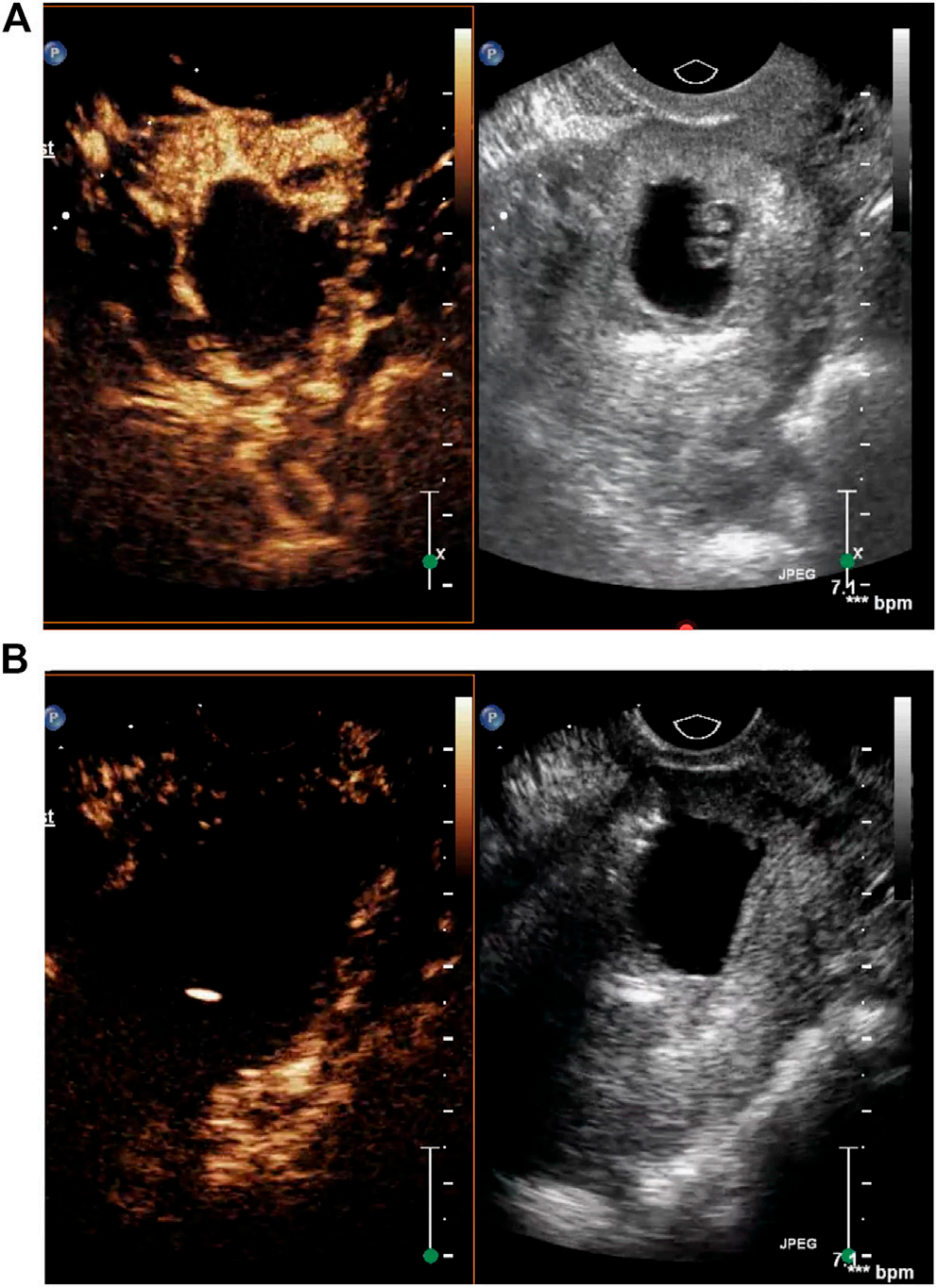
(A) The contrast-enhanced ultrasound picture indicated abundant blood supply in the area surrounding the gestational sac. (B) Transvaginal contrast-enhanced ultrasonography before curettage showed that the blood flow in the peripheral tissue of the pregnancy sac was completely occluded.
